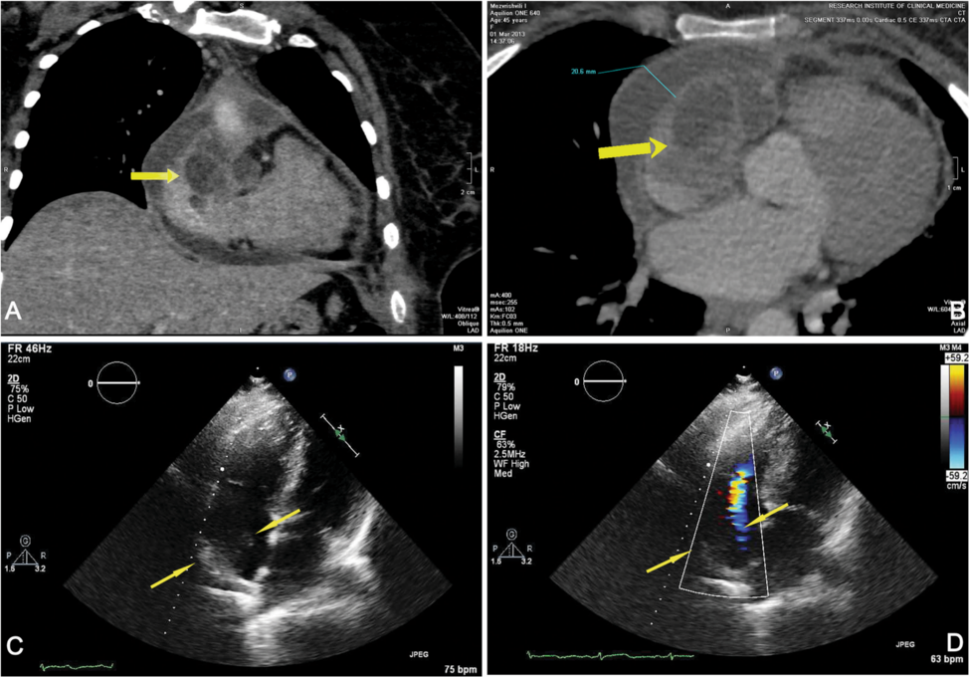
a, b Computed Tomographic (CT) coronary angiography. Low-density tumorous mass (50 mm in diameter) at the level of auricle of the right atrium. c, d Transesophageal echocardiography. Tumor in the auricle of the right atrium, spreading toward superior vena cava
